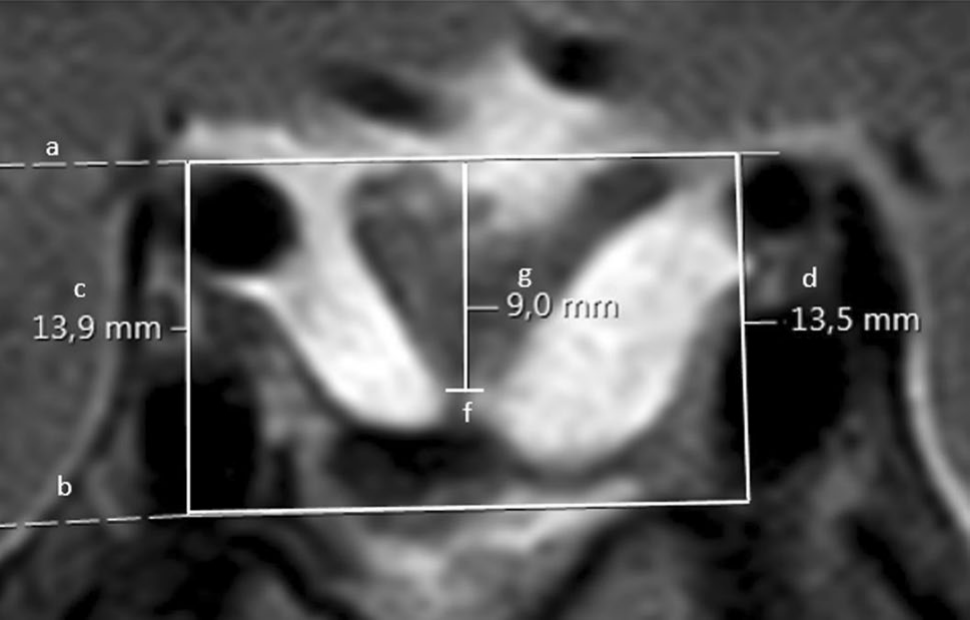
MRI scan demonstrating a downward displaced optic chiasm classified with the Chiasm Herniation Scale (CHS). Line (a) connects the superior border of the superior carotid arteries at their entry point into the cavernous sinus. Line (b) connects the inferior border of the inferior carotid arteries at their entry point into the cavernous sinus. The mean distance between those lines is (e) (e = 13.7 mm), extracted from (c) (c = 13.9 mm) and (d) (d = 13.5 mm). The lowest point of the optic chiasm is (f). The distance from (f) to line (a) is (g) (g = 9.0). The proportional herniation is calculated with (e) and (g). Example: $${\text{CHS }} = { 1}00 \, \times {\text{ g}}/{\text{e}}\quad {\text{e }} = \, \left( {{\text{c}} + {\text{d}}} \right)/{2}$$, $${\text{CHS }} = { 1}00 \, \times { 9}.0 \, /{ 13}.{7 } = { 65}.{7}\quad {\text{e }} = \, \left( {{13}.{9} + {13}.{5}} \right)/{2}$$,$${\text{CHS }} = { 65}.{7} \quad {\text{e }} = { 13}.{7}$$
